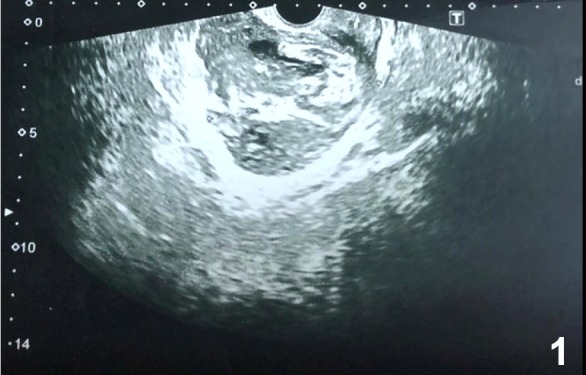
Pelvic ultrasonography showing an 8 cm diameter abscess.
